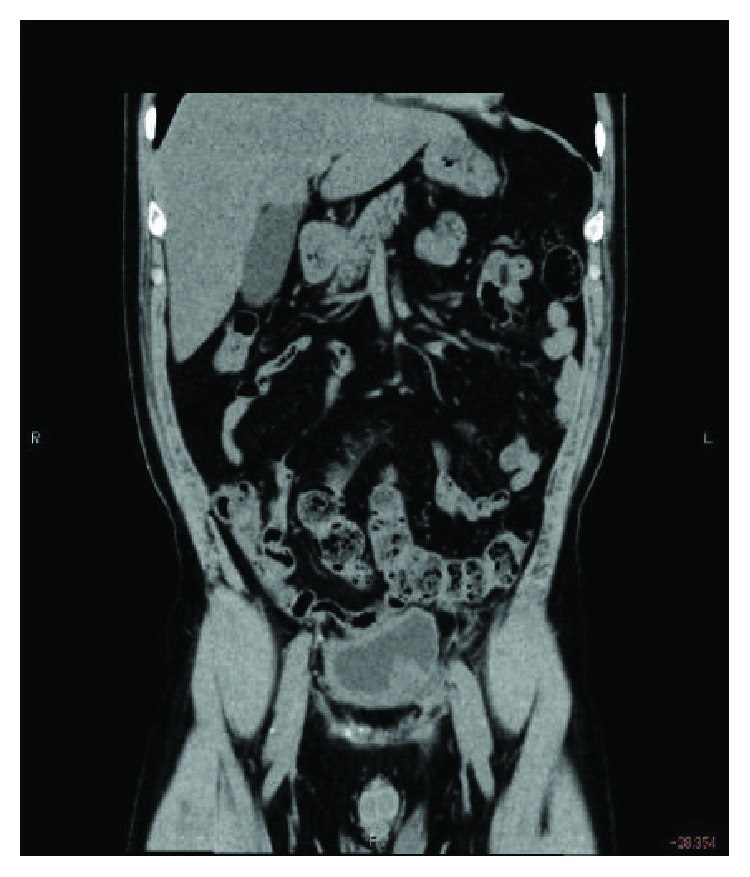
Postoperative CT findings. A coronal slice revealed that appropriate repair for bilateral bladder sliding hernia was performed.
